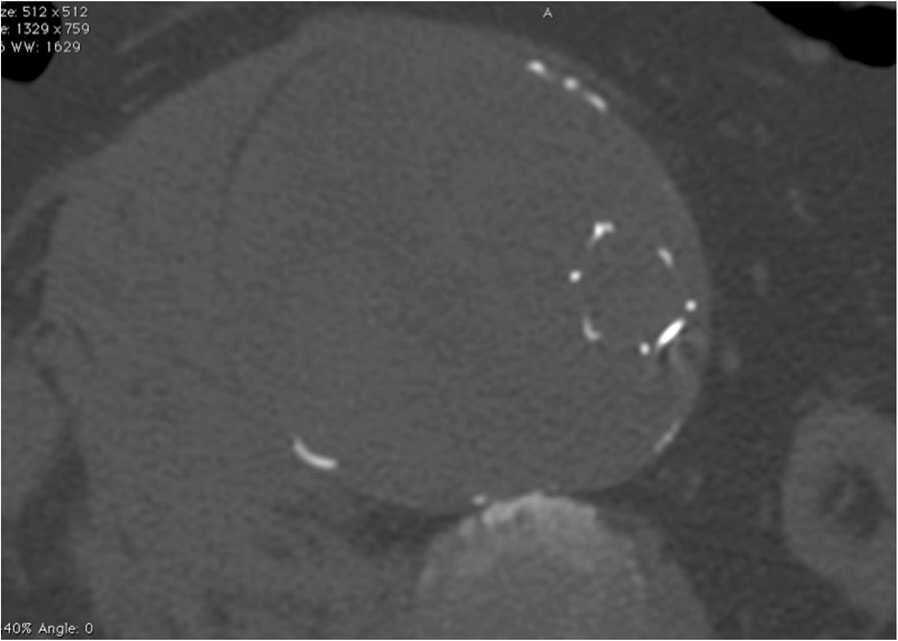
CT-scan without media-contrast, at Emergency room which showed a large increase of abdominal aortic diameter (140 × 130 mm) with rupture and hemoperitoneum. CT-scan: Computed Tomography scan
